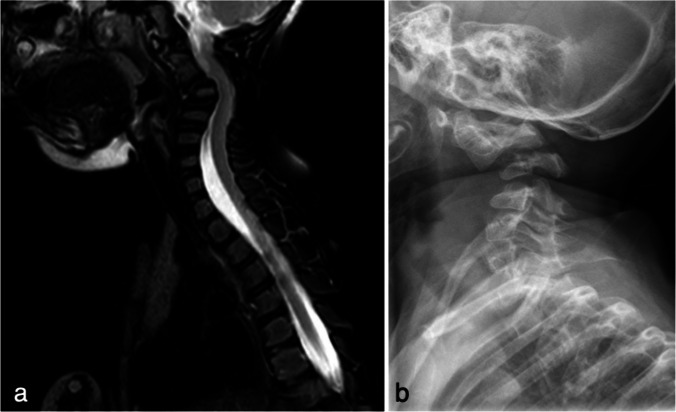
a Sagittal T2-weighted MRI at age 15 months reveals cervical kyphosis with ventral compression of the spinal cord by the hypoplastic C4 vertebral body. b Lateral cervical radiograph reveals the bony anomalies, underdeveloped vertebral, and indication of ligamentous laxity. The patient underwent C3 corpectomy with C2–C4 anterior and posterior fusion and was previously reported [10]
